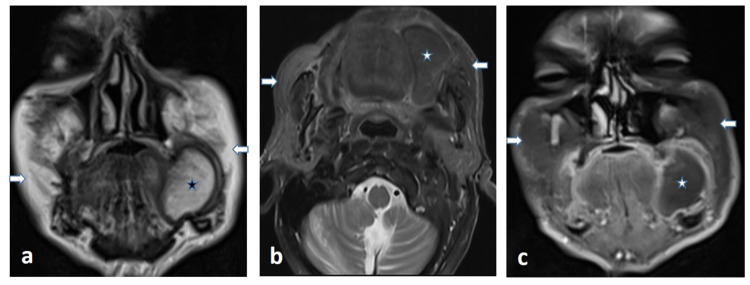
MRI images of the maxillofacial region. In the coronal T2W image (a) the well-circumscribed mass lesion (asterisk) in the right buccal region is completely isointense to the subcutaneous fatty tissue (arrows). Axial fat-suppressed T2W image (b) demonstrates total signal loss in both the lesion (asterisk) and also subcutaneous fatty tissues (arrows) which is consistent with the mass’ lipomatous nature. Contrast-enhanced coronal fat-suppressed T1W image (c) shows that there isn’t any enhancement of the lipomatous mass except for its peripheral thin smooth capsule. Lipoma is diagnosed as per the findings. T2W: T2-weighted; T1W: T1-weighted
